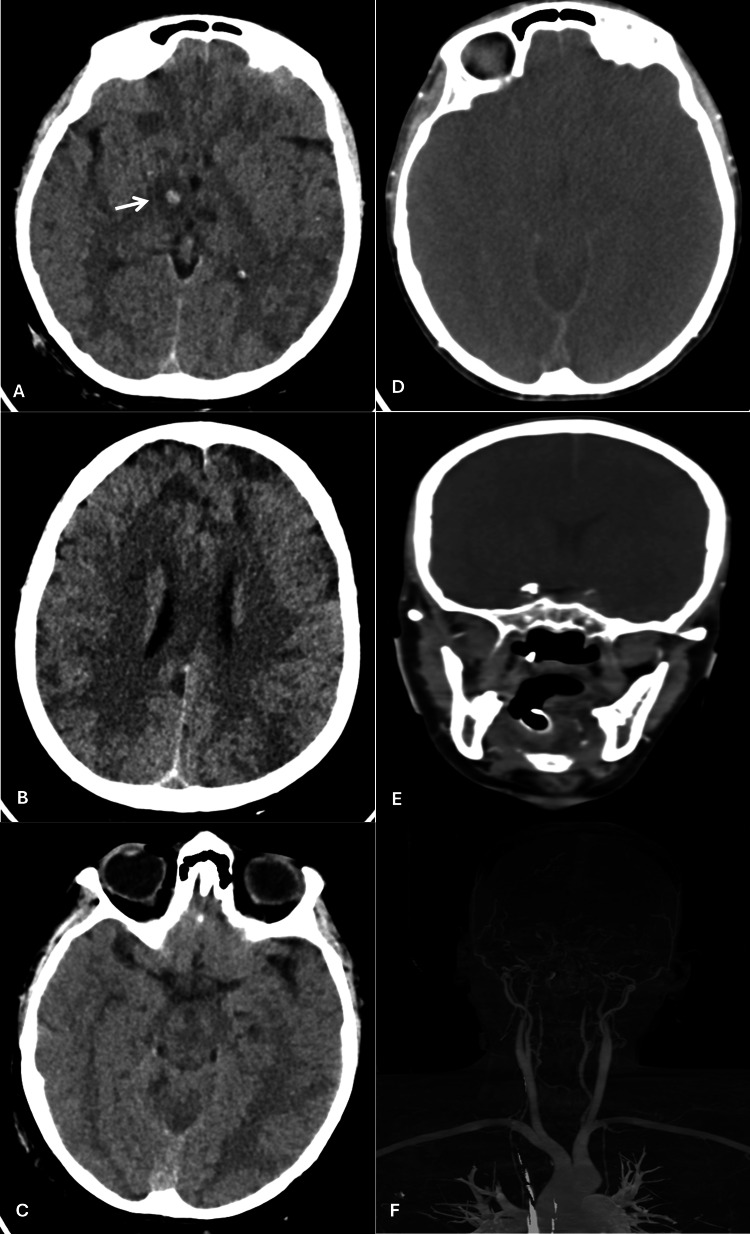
Computed tomography (CT) of the brain (A) Axial non-contrast CT showing a right basal ganglia hemorrhage with surrounding edema, causing effacement of the third ventricle.
